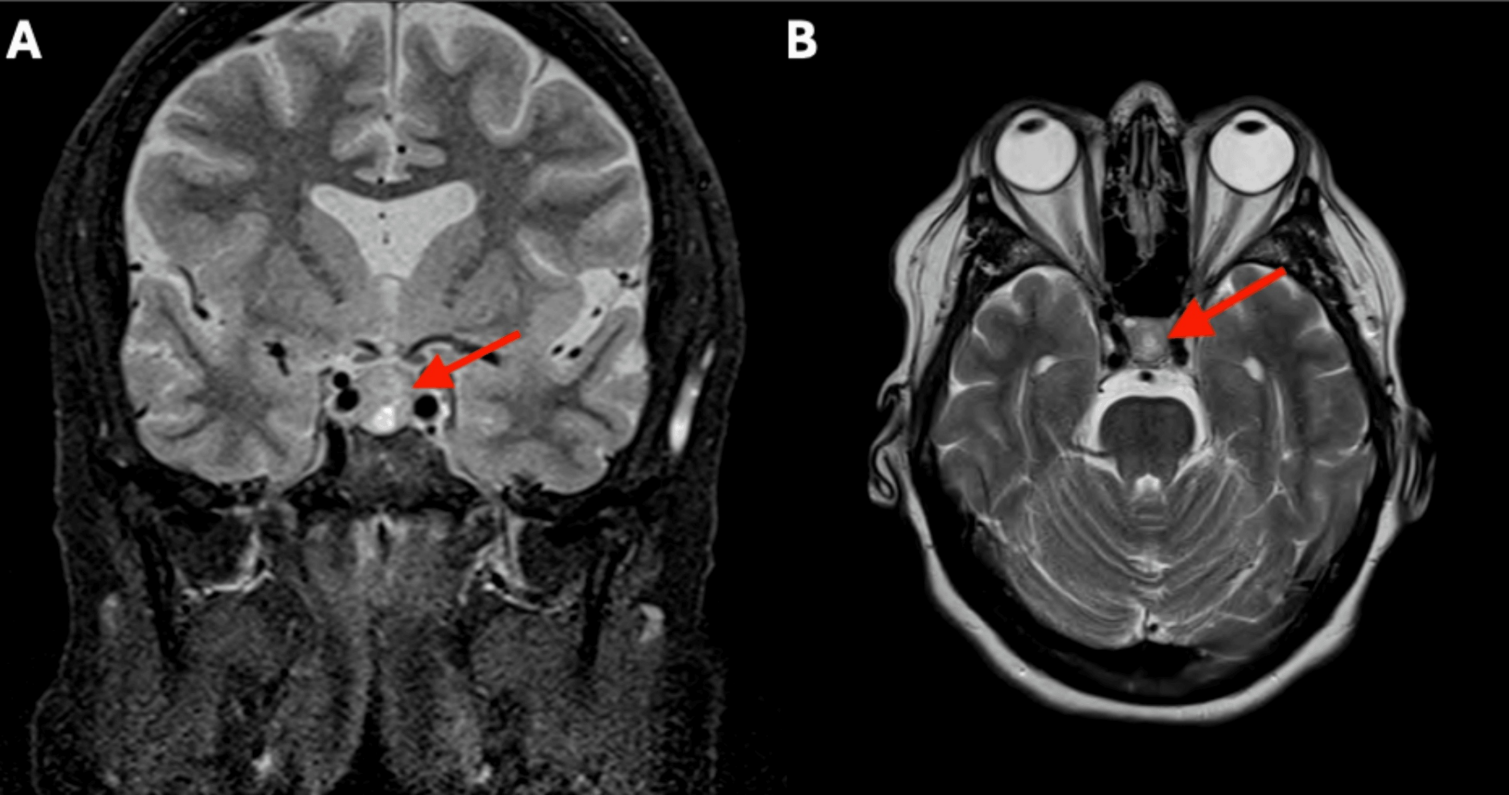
Coronal (A) and axial (B) T2-weighted MRIs showing NF-PitNET prior to resection The red arrow indicates the NF-PitNET, measuring 1.2 cm × 1.5 cm. NF-PitNET, nonfunctioning pituitary neuroendocrine tumor
